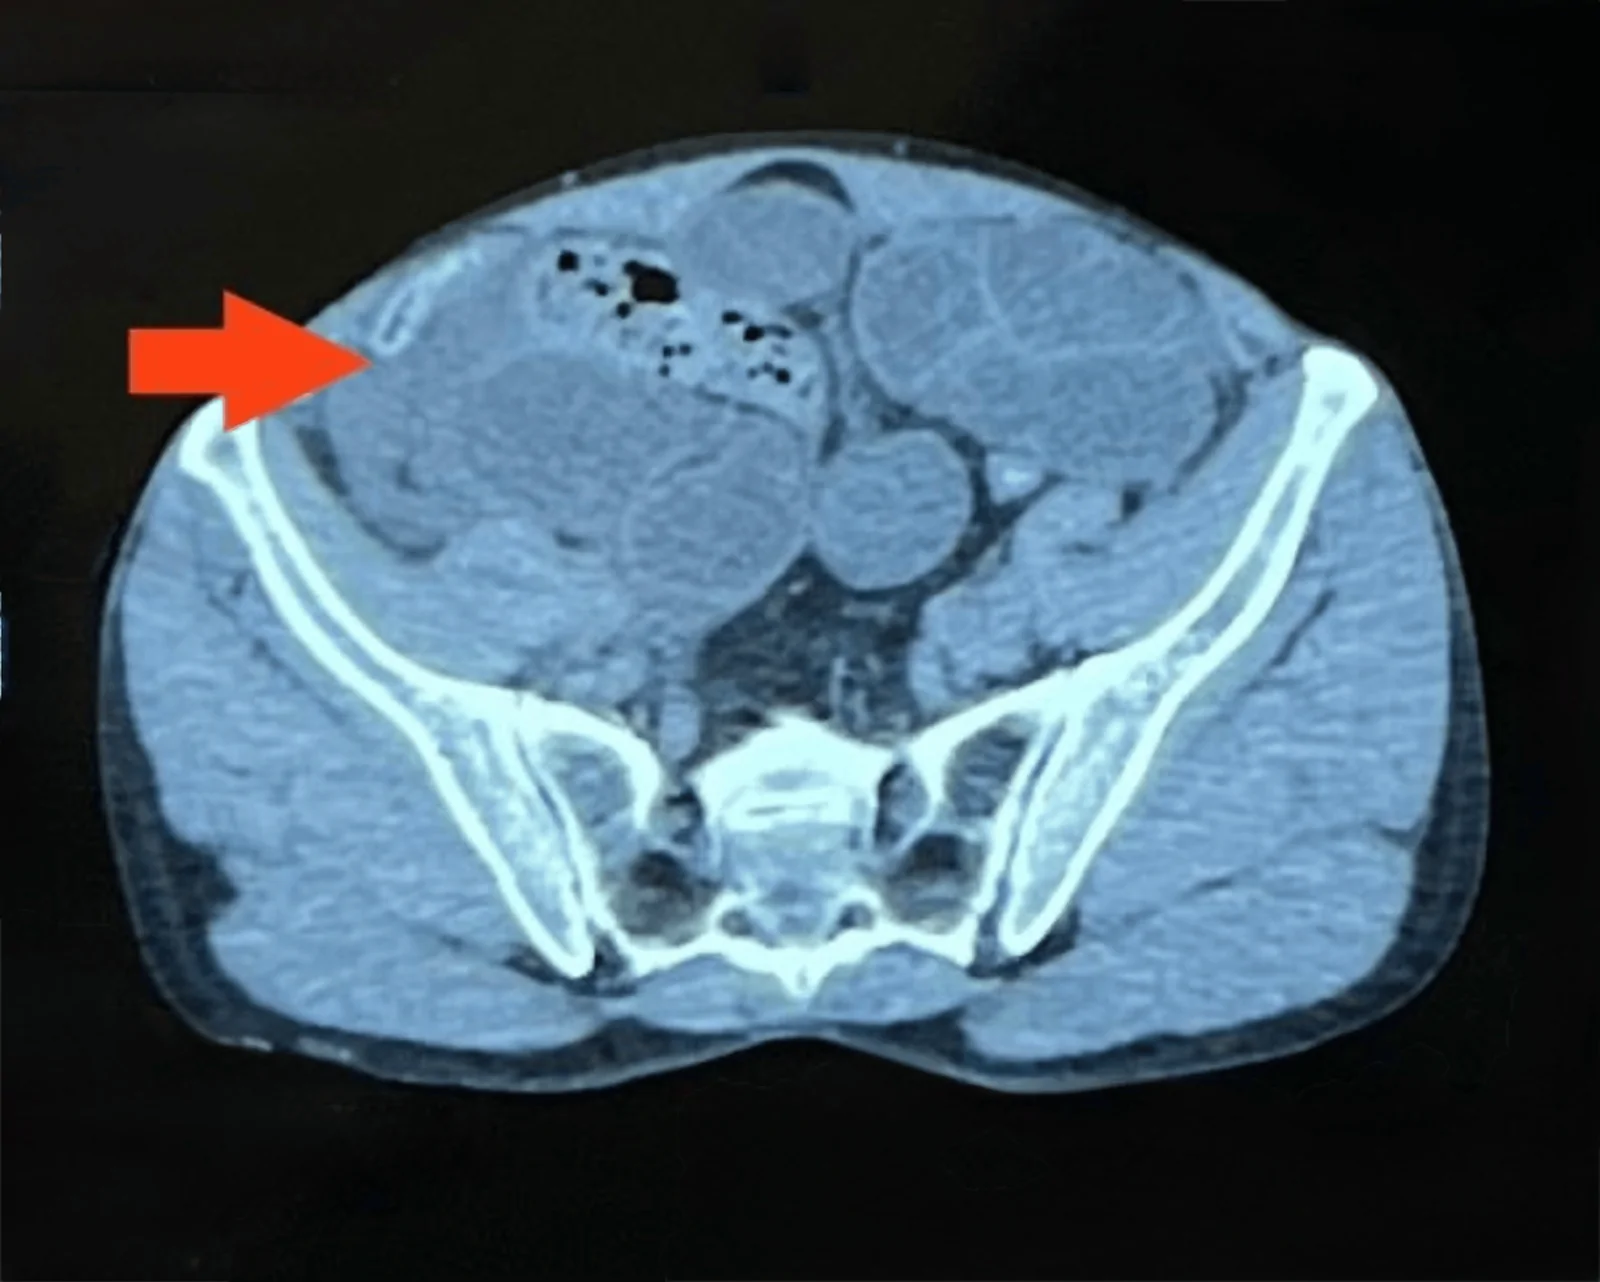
Computed tomography (CT) scan showing small bowel obstruction
